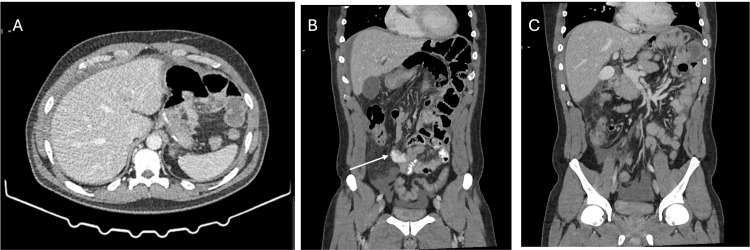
Contrast-enhanced abdominal and pelvic CT scan. Axial (A) and coronal (B and C) views demonstrating perihepatic, paracolic, and pelvic free fluid without evidence of pneumoperitoneum or pneumatosis intestinalis. A subtle mesenteric “swirl sign” is visible (arrow), raising suspicion for an internal hernia and prompting surgical exploration.
